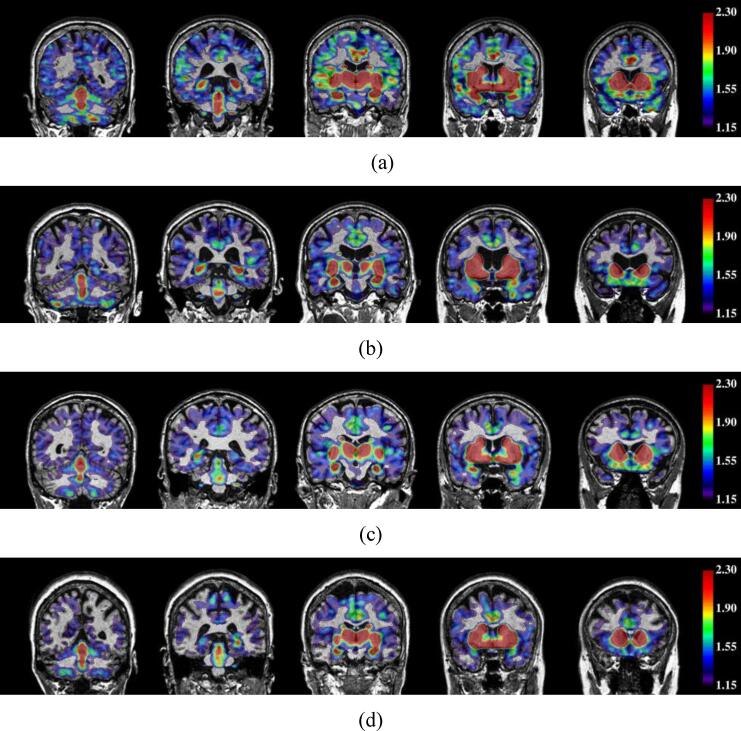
Example FEOBV SUVR images overlayed on structural MR images with global cortical FEOBV SUVR = 1.417, 1.268, 1.256 and 1.185 respectively for (a) Aβ- CU, (b) Aβ+ CU, (c) Aβ- MCI and (d) Aβ+ MCI participants. Regions without the overlaid colour indicate the FEOBV SUVR values to be < 1.15.
